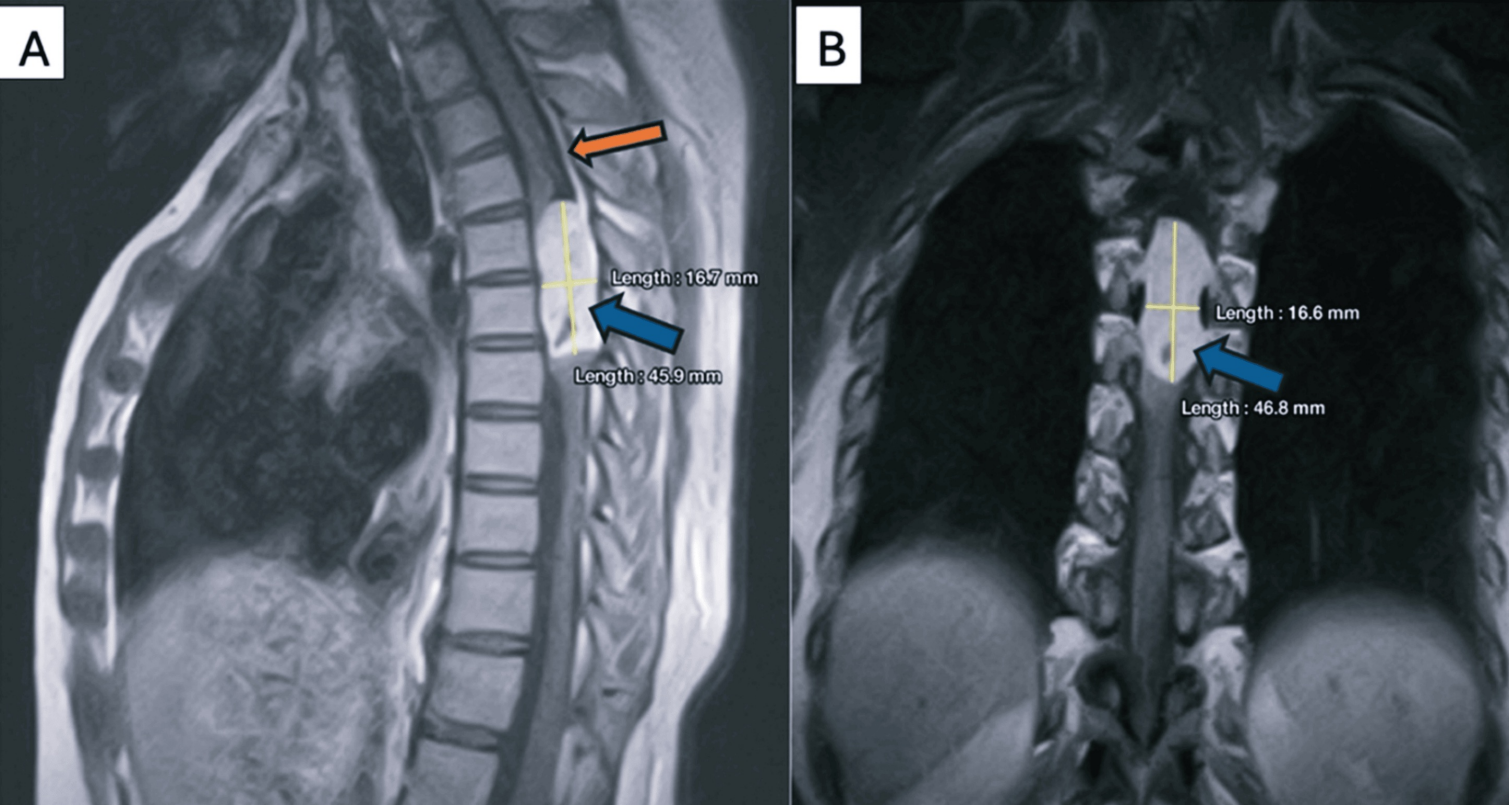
Thoracic MRI with gadolinium contrast. Sagittal (A) and coronal (B) views showing a homogeneously contrast-enhancing lesion over T4-T5 vertebrae (blue arrows) measuring 45.9×16.7×16.6 mm. Dural thickening (dural tail) is visible on the superior border of the tumor (orange arrow). The tumor fills the spinal canal completely, compressing the spinal cord.
